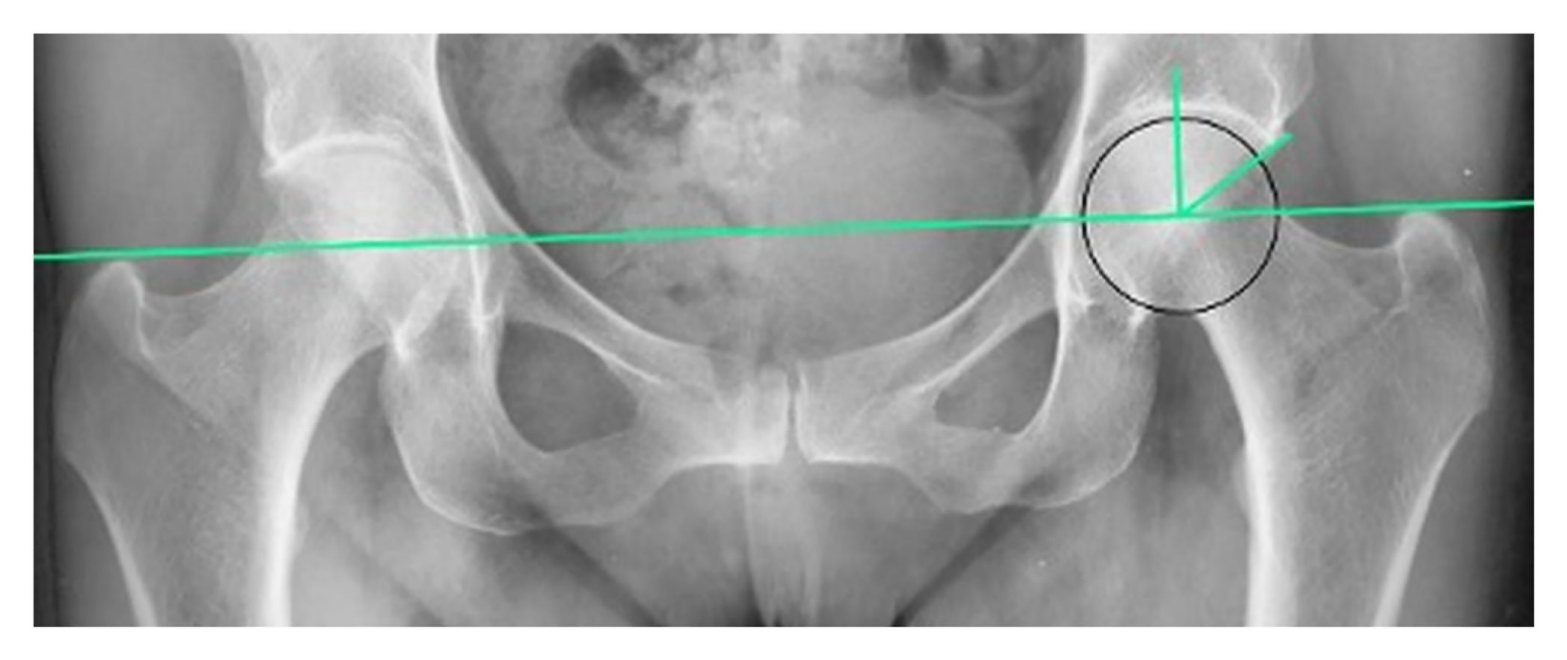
The lateral centre edge angle (LCEA) is measured on an anteroposterior (AP) pelvic radiograph. The LCEA was constructed according to the following steps. A horizontal reference line was constructed to correct for pelvic tilt in the radiograph and was based on the average of four lines, between (1) both femoral head centres, (2) the most cranial points of the foramen obturator, (3) the most caudal point of the ischial tuberosity and (4) the most caudal point of the pelvic teardrop. To determine the centre of the femoral head, a best fitting circle was drawn around the femoral head based on the statistical shape modeling (SSM) points. The LCEA was then formed by two lines drawn from the centre of the best fitting circle. The first line was drawn vertically through the centre of the femoral head, perpendicular to the horizontal reference line. The second line was drawn from the centre of the best fitting circle to the most lateral bony point of the acetabulum.
